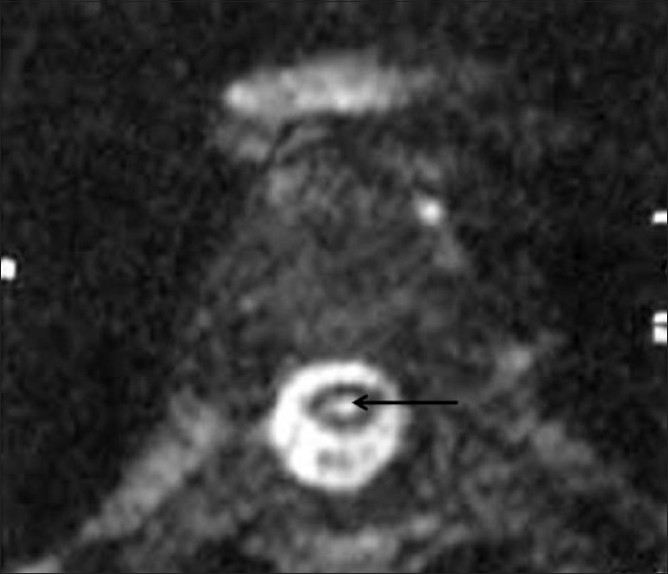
Axial T2W MRI image of the cervical cord better demonstrates the central cervical cord hyperintensity (arrow)
